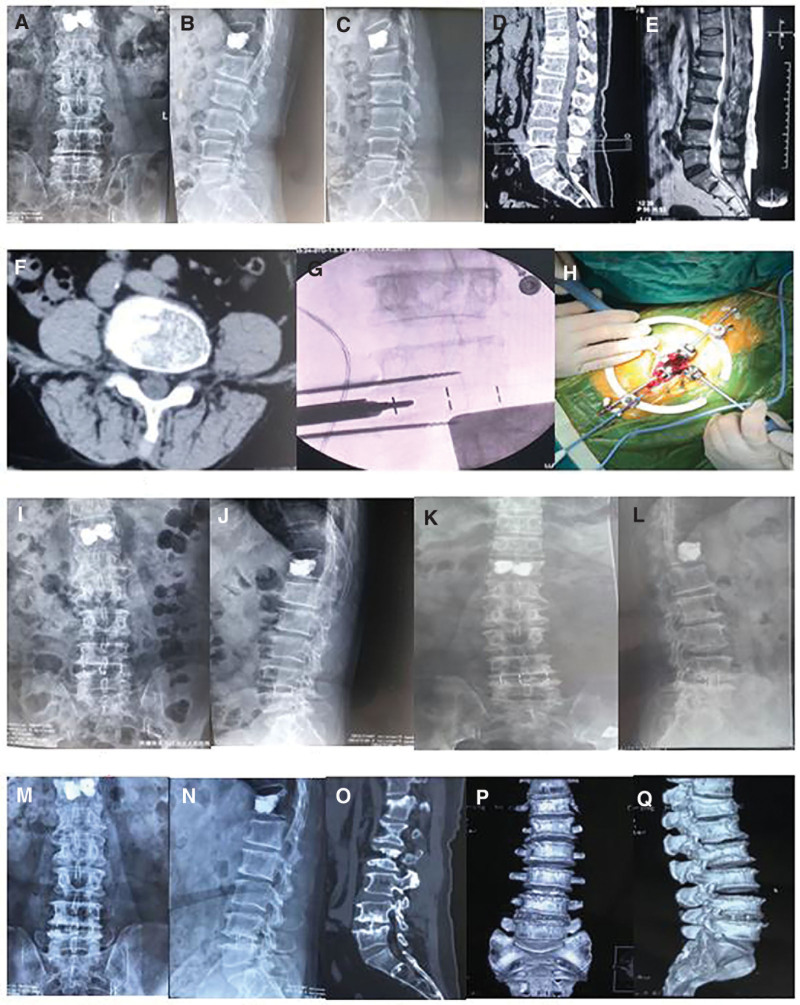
The patient was a 47 year old male, with low back pain and limited activity, without neurological symptoms of both lower limbs. His preoperative imaging examination (A–F), and He chosed CLIF operation (G, H). He underwent X-ray examination in 3 (I, J), 6 (K, L)and 12 (M–O)months after operation, and CT 3-dimensional reconstruction in 6 months after operation (P, Q). CLIF = Crenel lateral interbody fusion.
